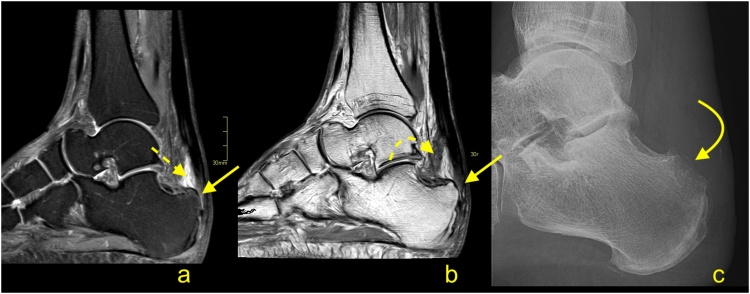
Chronic complication after surgery for Haglund’s syndrome. A 72-year-old patient presented 3 months after surgery for Haglund's syndrome with pain over the Achilles tendon insertion. The postoperative MRI (a and b) showed thinning of the Achilles tendon insertion (straight arrow), fluid in the retrocalcaneal bursa (curved arrow) and adhesions in Kager's fat pad (dashed curved arrow). Two months later, the patient presented with heel pain and reddening of the skin. Clinical suspicion of osteitis was confirmed on the X-ray (c), where osteolysis can be seen in the supero-posterior part of the calcaneus (curved arrow). A- T2- weighted with fat suppression, b- PD- weighted, c- the lateral projection of the calcaneus.
